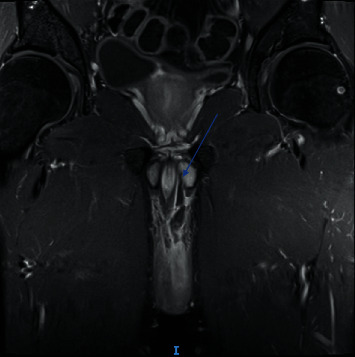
T1-weighted venous time fontal pelvis MRI, focal tear of the medial aspect of the left corpora, adjacent to the penile bulb.
